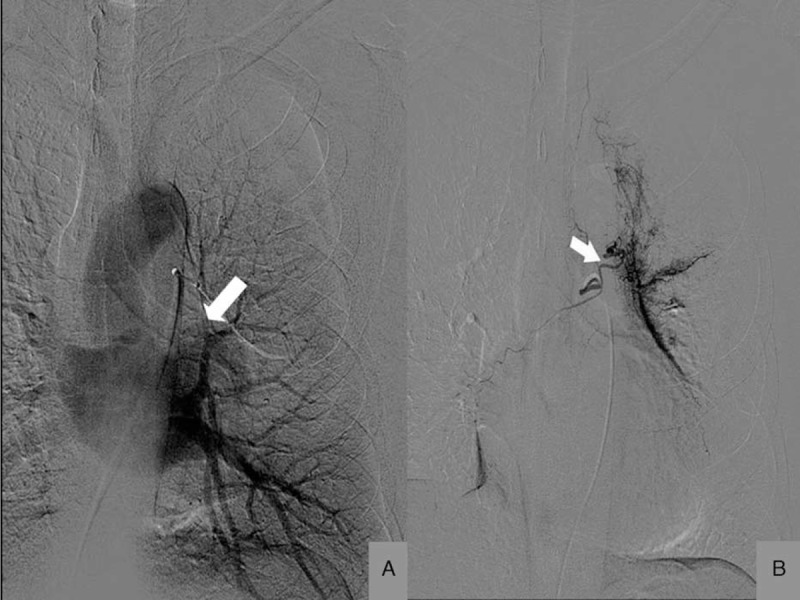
Pulmonary angiography for pulmonary vein stenosis (PVS) patient. (A) Case 5: pulmonary angiography shows an occlusion of left superior pulmonary veins. (B) Case 5: bronchial artery radiography shows that the bronchial artery was dilated.
